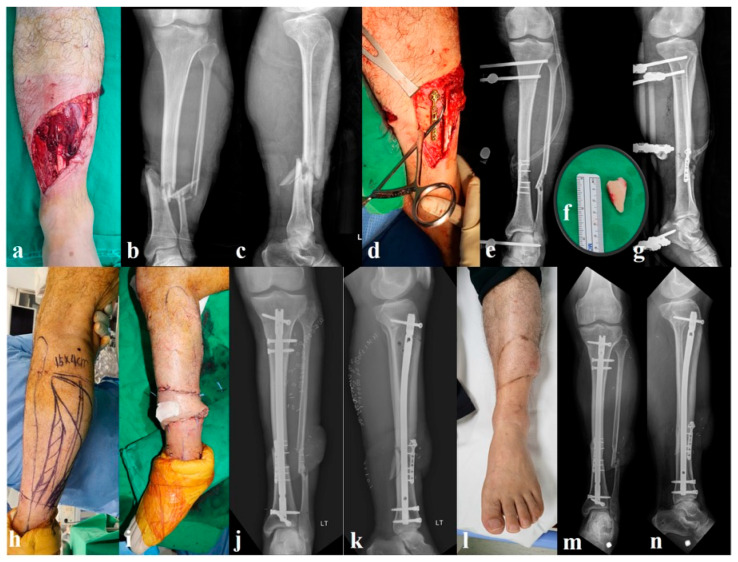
A 37-year-old man who sustained a Gustilo–Anderson type IIIB open tibial shaft fracture (Arbeitsgemeinschaft für Osteosynthesefragen/Orthopaedic Trauma Association 42-B) following a motorcycle accident (a–c). After irrigation and debridement, provisional reduction was achieved using a 2.7 mm miniplate (d) and stabilized with an external fixator (e). A free wedge fragment measuring 3 × 1.8 cm (f) was removed during debridement due to loss of viability. Definitive fixation with intramedullary nailing and rotational flap coverage was performed simultaneously (h–k). The wound healed uneventfully (l), and final follow-up radiographs showed complete bone union without additional bone grafting (m,n).
